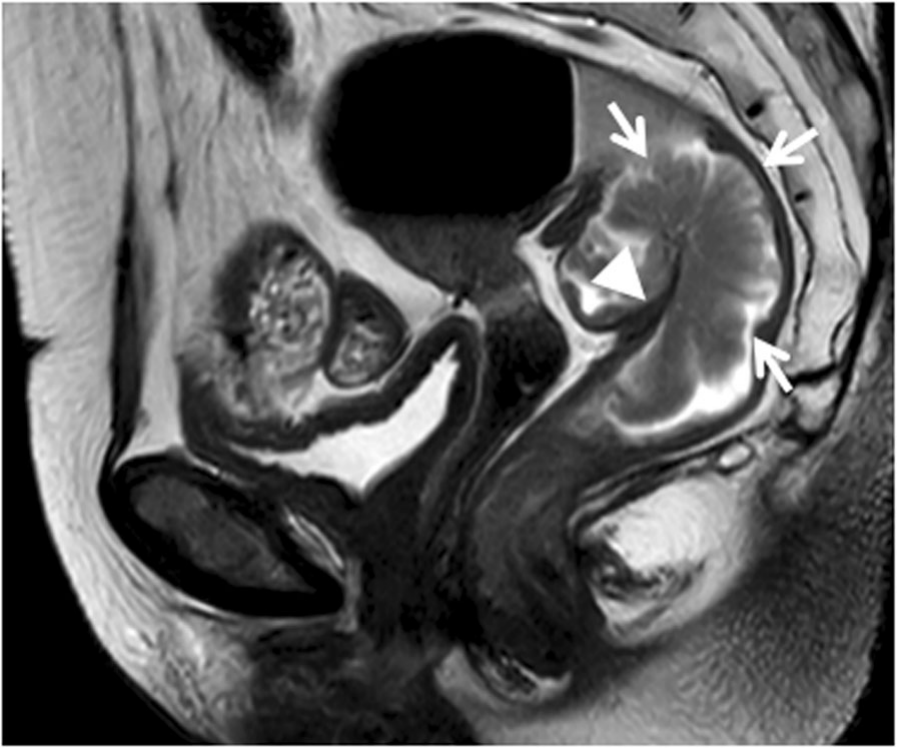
Sagittal T2-weighted imaging of a rectal cancer (intermediate signal intensity) presenting as polypoid mass (arrows) protruded into lumen with distinct intestinal wall (arrow head). Result of the postoperative pathology confirmed that the tumor invaded the submucosa without extending into muscularis propria
